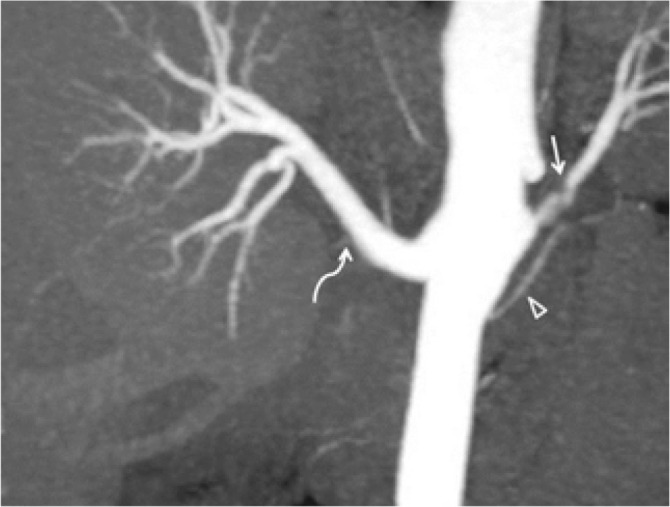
Maximum intensity projection coronal image from CT Angiography depicts stenosis in the main renal artery (arrow) and patent aberrant renal artery (arrowhead) on left along with normal calibre right renal artery (curved arrow).
